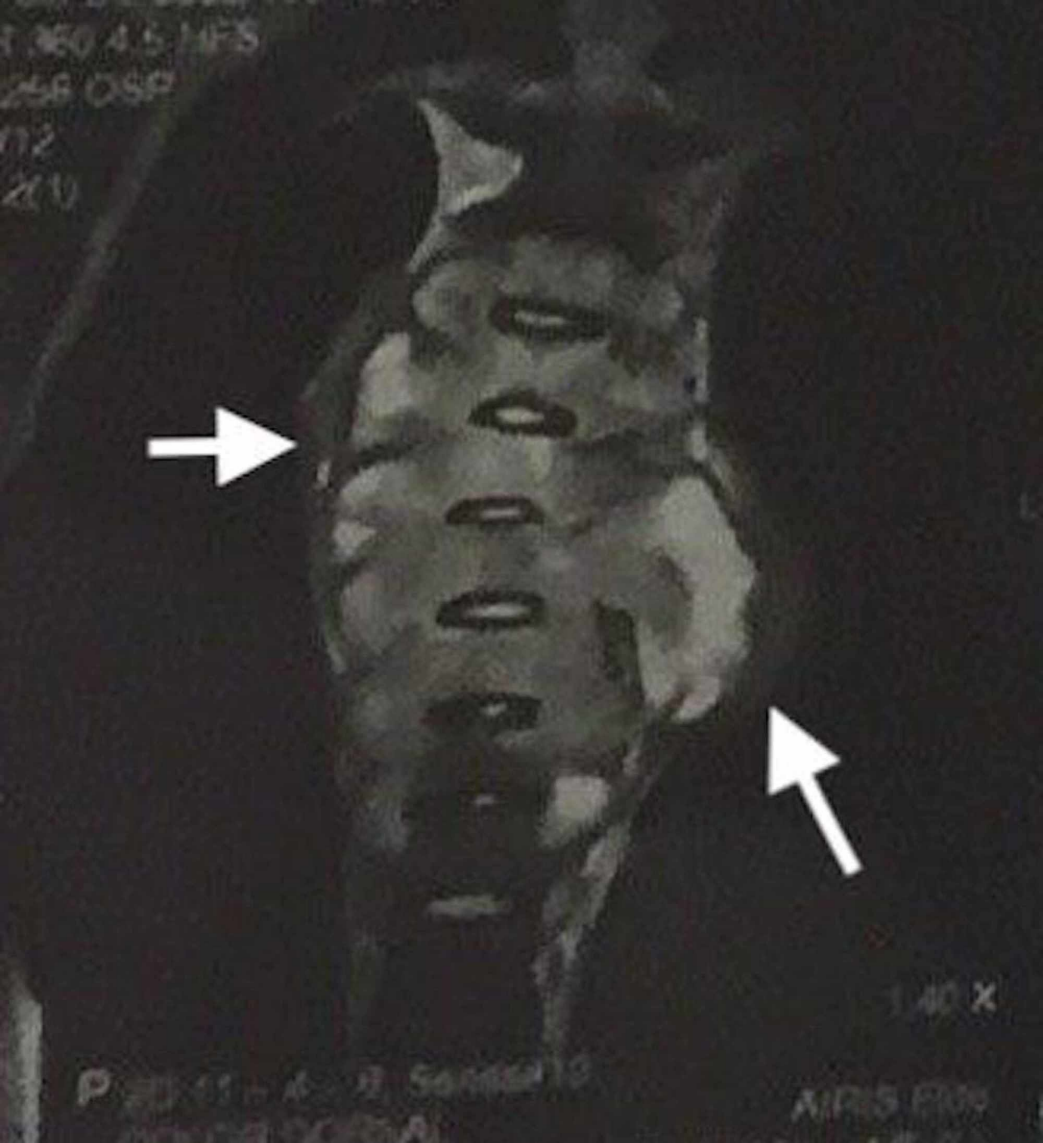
MRI coronal view Showing large paravertebral lesion with cystic appearance and an infiltration of the vertebral bodies.
